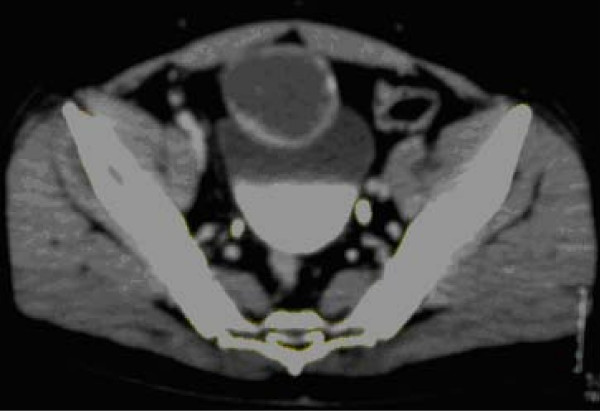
Contrast-enhanced CT scan at level of iliac crests shows a low attenuation cyst overlying the anterosuperior portion of bladder with focal calcifications.
